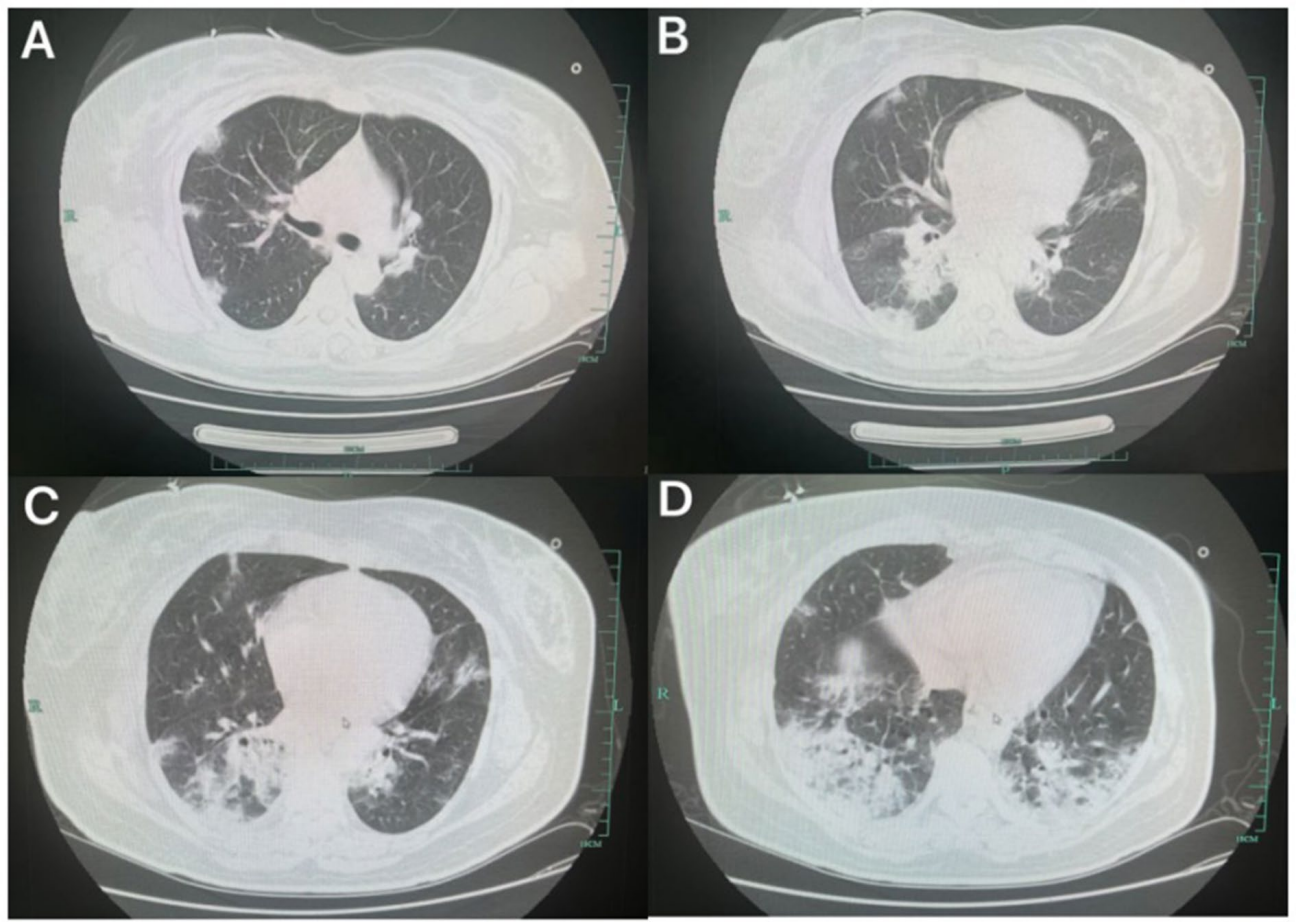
Lung CT (A–D) at the time of admission, showing ground-glass opacities in both lungs.
